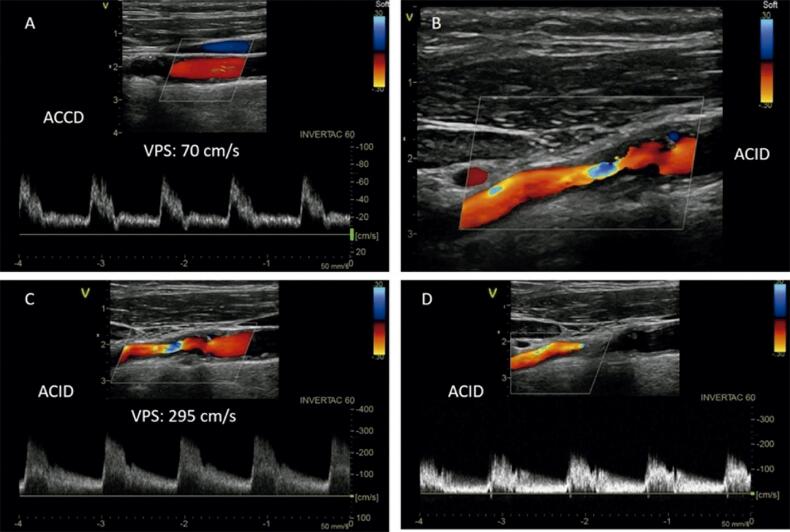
Estenose entre 70 a 79% da artéria carótida interna. A) Fluxo da artéria carótida comum; B) Estenose da artéria carótida interna ao Doppler colorido; C) Fluxo da artéria carótida interna no ponto da estenose; D) Fluxo turbulento pós-estenótico na artéria carótida interna.
